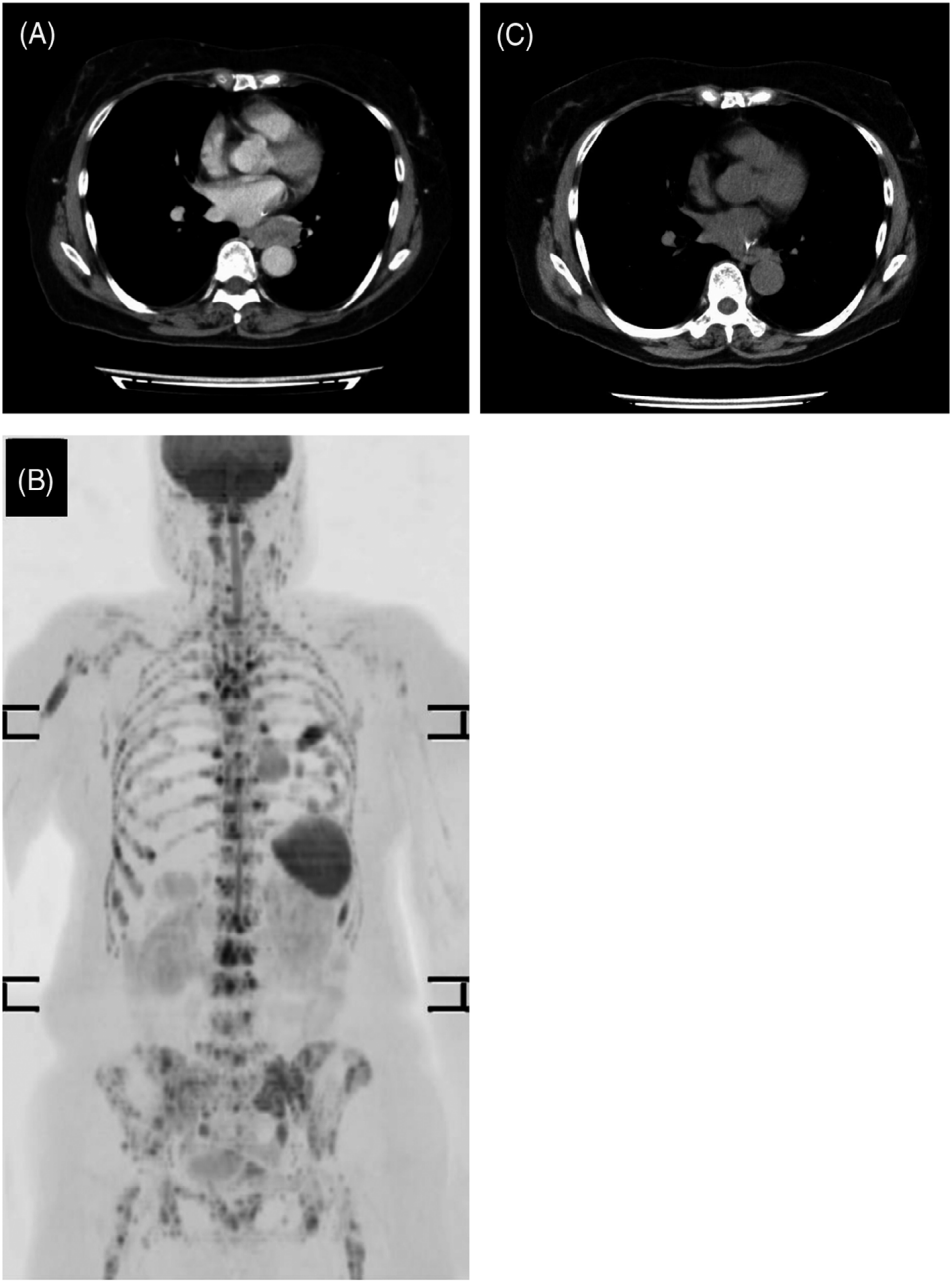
Chest contrast computed tomography (CT) at initial examination showing a substantial mass with slight enhancement in the lower lobe of the left lung (A). Whole‐body magnetic resonance diffusion‐weighted image showing systemic bone metastasis and lung tumour (B). Chest contrast CT 2 years after the initial examination showing shrinking of the tumour (C)
